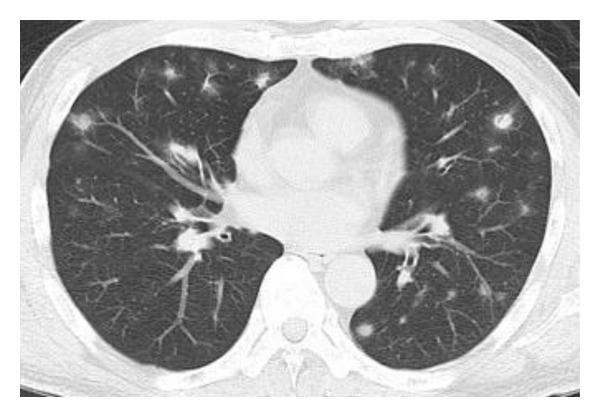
A chest computed tomography showed bilateral multiple nodules predominantly subpleural parenchyma.
